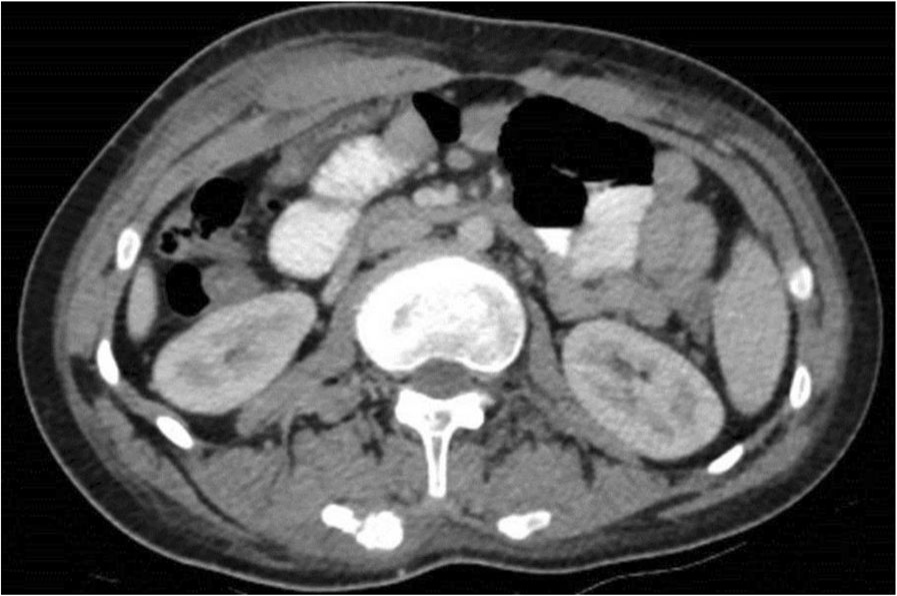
Coarse “calcifications” within lumbar paravertebral muscles on total body CT
